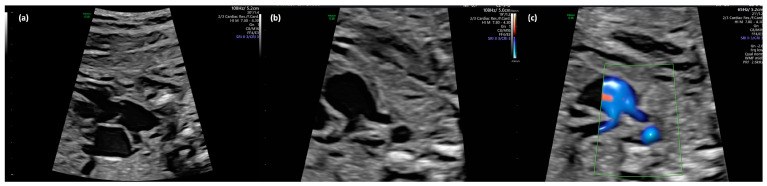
(a–c): Truncus arteriosus type 1 at 22 + 0 weeks in B-mode (a,b) und with color doppler (c).
